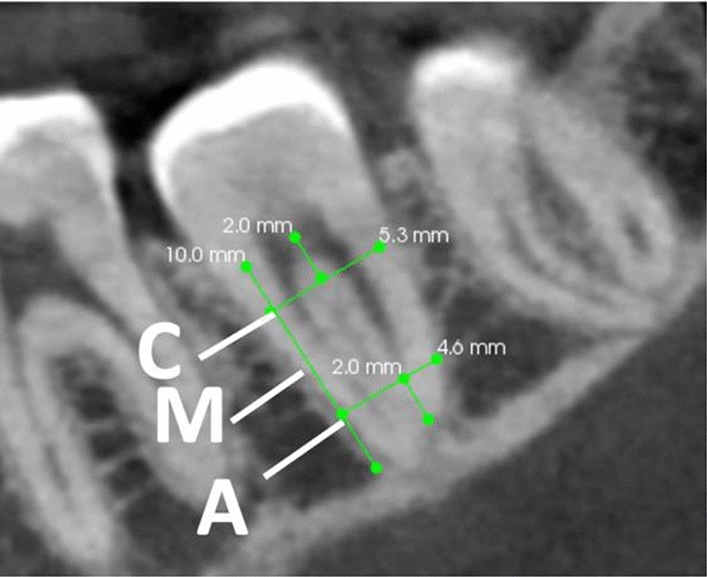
Representative sagittal CBCT view of a mandibular second molar generated by oblique slicing module. Exemplifying the three axial levels i.e., coronal (C), middle (M), and apical (A) at which the evaluation was performed.
